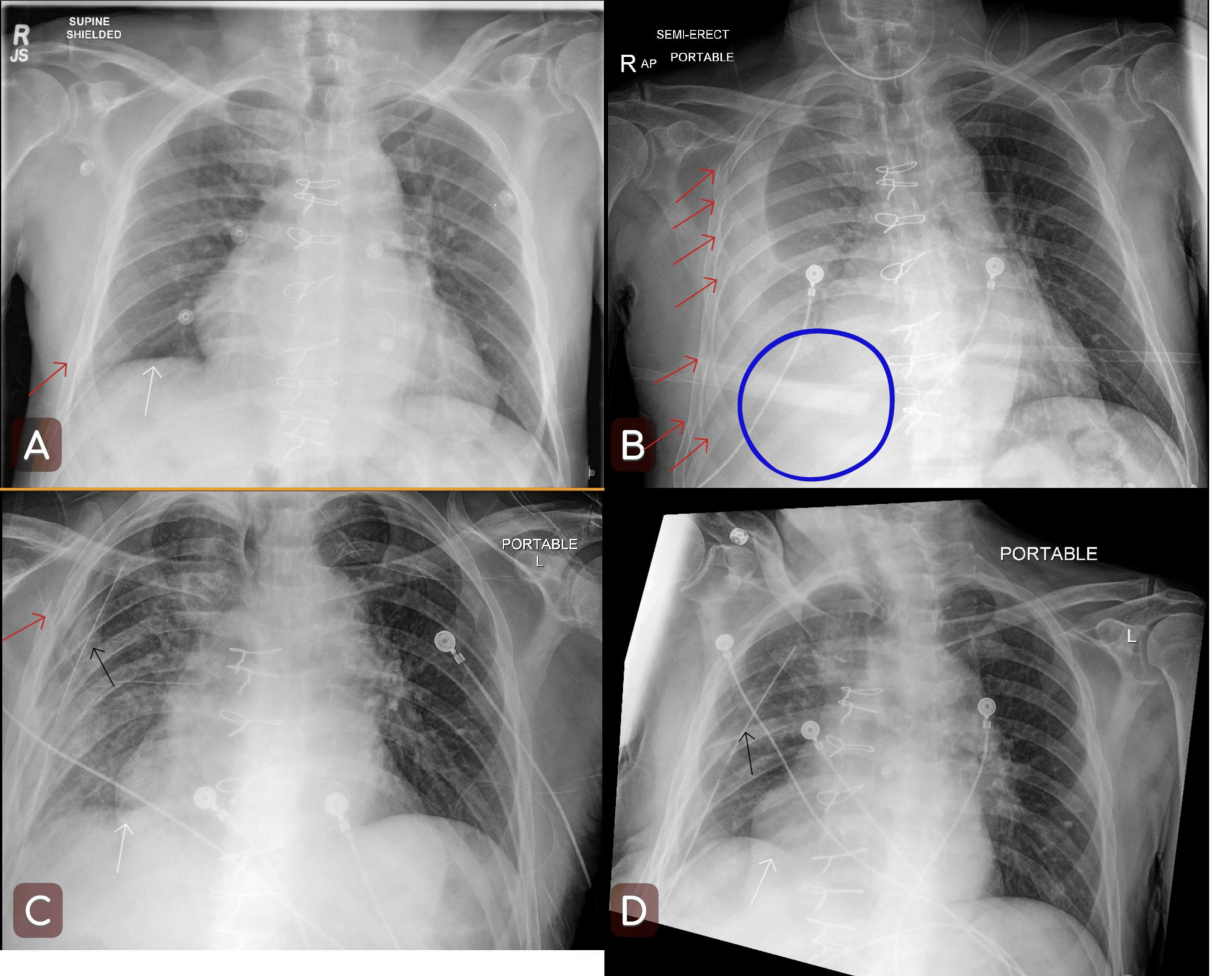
Serial Chest X-rays of the Patient Chest X-rays taken during the first ED visit, second ED visit, post-op day 1, and post-op day 8 (A, B, C, D). Rib fractures are marked with red arrows. Diaphragm outlines are marked with white arrows. Opacities in panel B representing pleural effusion are marked with a blue circle. The chest tube is marked using black arrows. Right lung borders in panel B are collapsed compared to other panels in the thorax, suggesting atelectasis.
